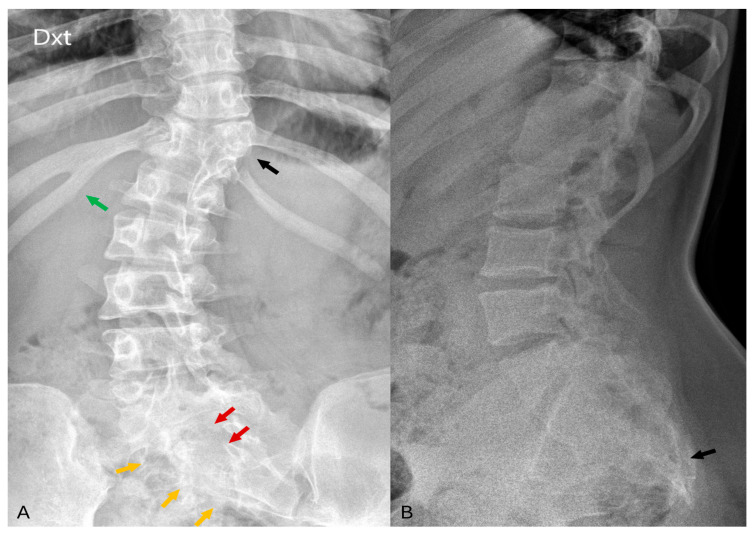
(A) In the AP projection of the lumbar spine radiographs, notable findings include right-sided fusion of the 11th and 12th ribs (green arrow), partial segmentation of the 11th thoracic vertebra (black arrow), and posterior arch defects observed in the sacral vertebrae (red arrows). Suspicious structural changes in the right side of the sacrum can also be visible (orange arrows). (B) In the LL projection of the lumbar spine, visualization reveals a deformed and shortened sacrum as well as coccyx agenesis (black arrow).
